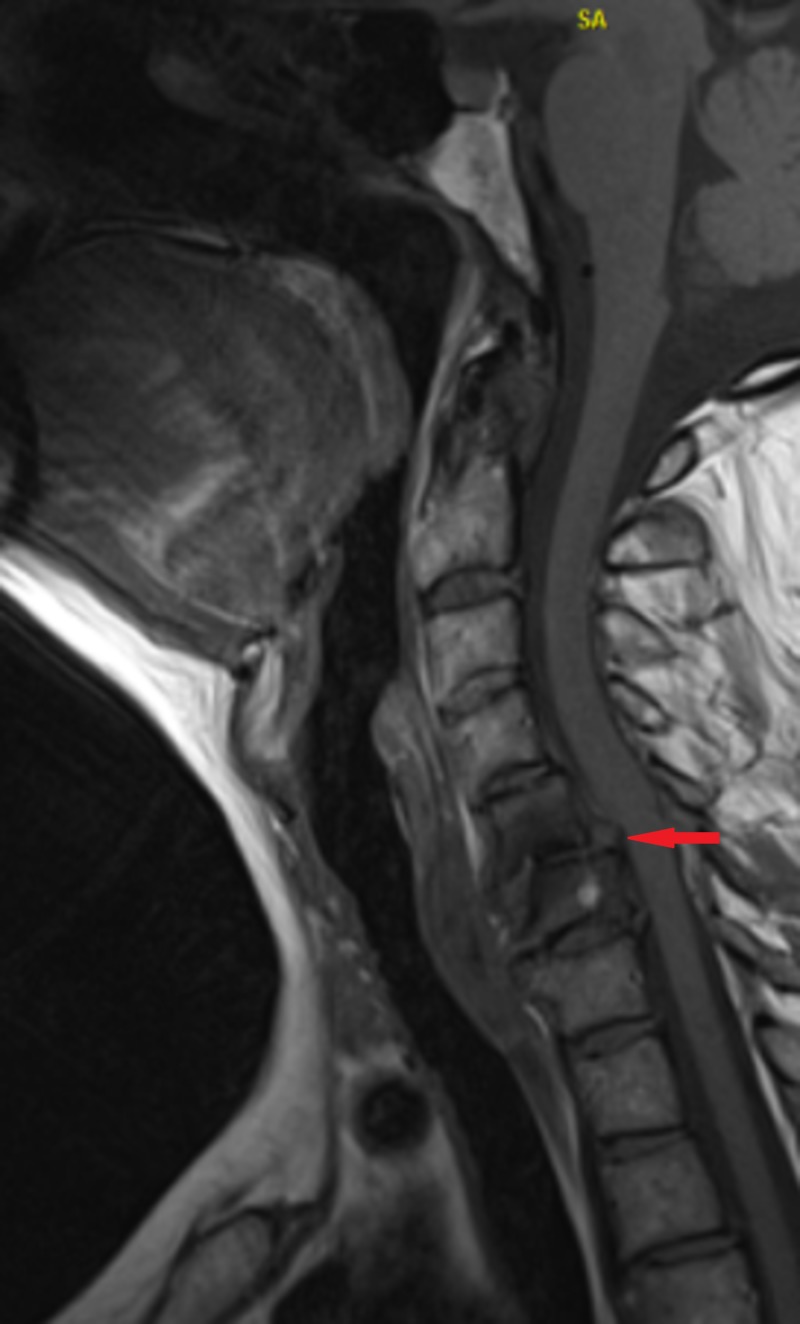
Magnetic resonance imaging of the cervical spine showing evidence of lower cervical spondylosis
